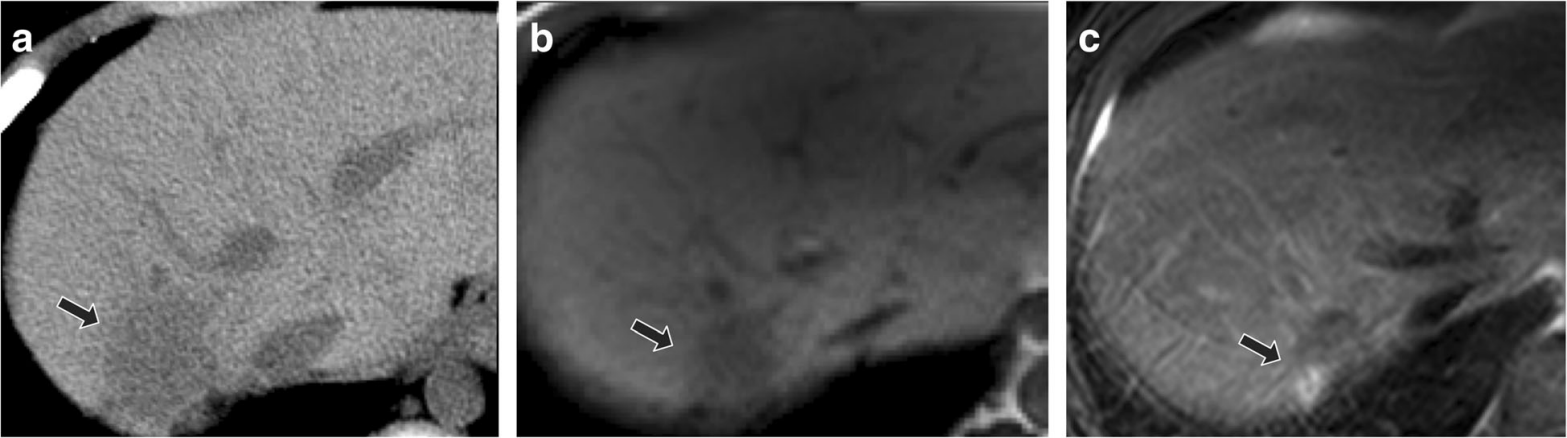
Focal Gaucher cell accumulation in the liver. Axial non-contrast abdominal CT (a) demonstrates a focal hypoattenuating hepatic lesion (arrow) in the posterior right hepatic lobe in 10-year-old male with type 1 Gaucher disease. At follow-up at 16 years of age with unenhanced MRI, lesion (arrow) was unchanged in size with T1-weighted hypointense (b) and mixed T2-weighted signal intensity (c). Findings are most consistent with focal Gaucher cell deposition (“Gaucheroma”)
